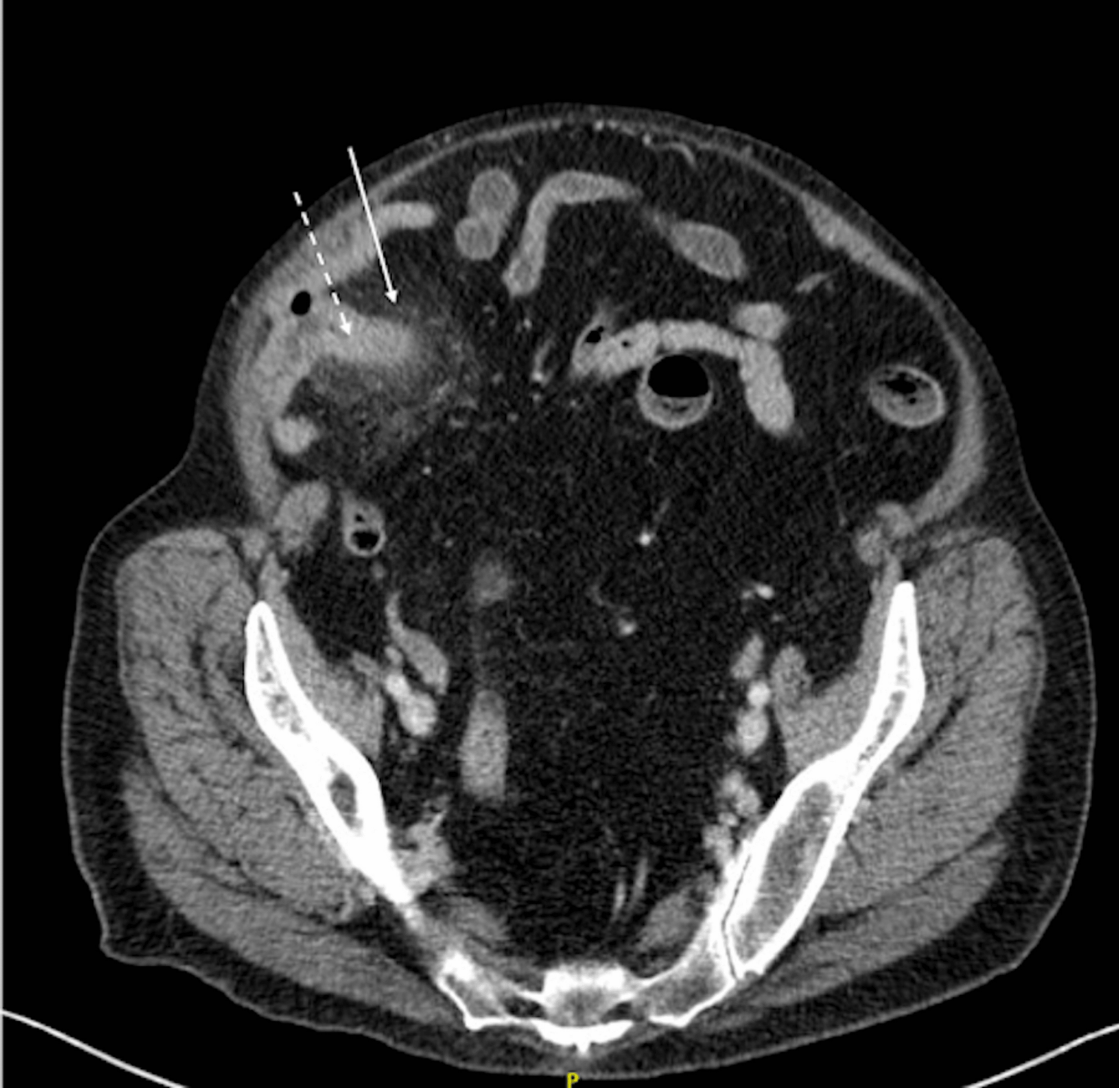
Axial plane of CT scan of abdomen and pelvis with IV contrast showing the appearance of an abnormally distended appendix (dotted white arrow) and abnormal fat stranding in adjacent soft tissues (white arrow). CT: Computed tomography; IV: Intravenous
